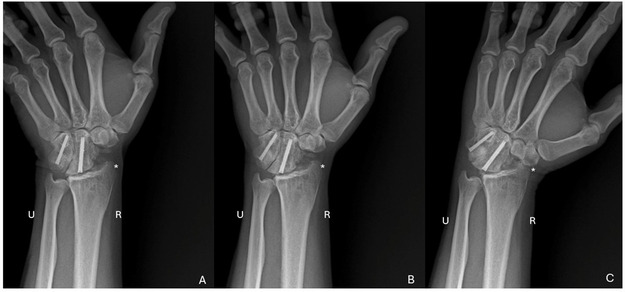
Left, supine on hand table in wrist traction tower. Preoperative dynamic wrist radiographs showing prominent radial styloid (*) impingement in (A) ulnar deviation, (B) neutral, and (C) radial deviation. (R, radial; U, ulnar.)
